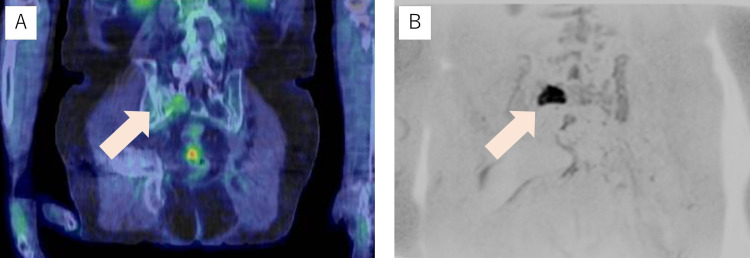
Coronal section image of the right sacral lesion (A) Coronal section image of the right sacral lesion in 18F-fluorodeoxyglucose positron emission tomography merged with computed tomography (arrow). (B) Coronal section image of the right sacral lesion in diffusion-weighted whole-body imaging with background body signal suppression (arrow).
